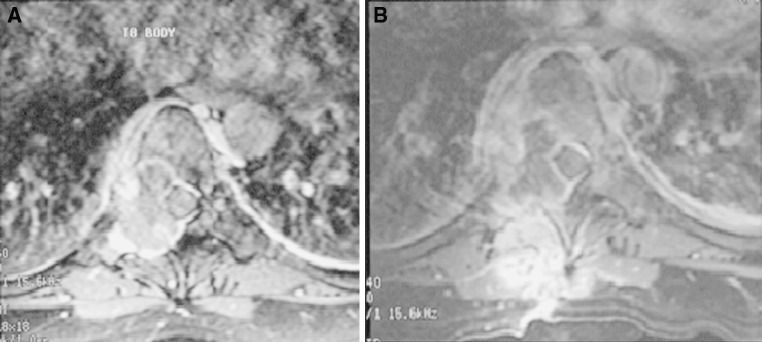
Paradoxical response after anti-TB treatment. a Contrast MRI at presentation, b contrast MRI after four weeks of anti-TB medications showed increased inflammation, soft tissue swelling and abscesses
